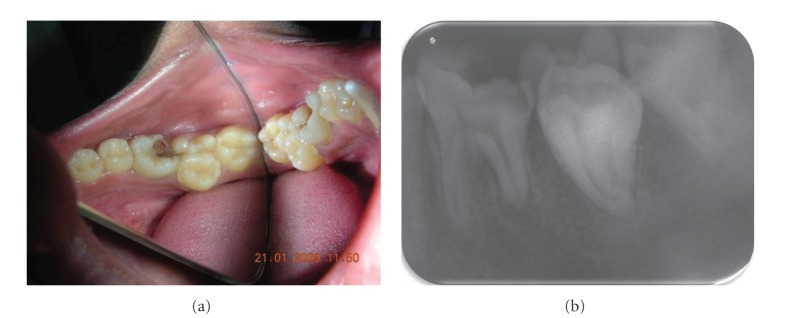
(a) Pre-operative photograph: Occlusal View. (b) Pre-operative radiograph revealing irregular morphology of tooth #20 & istal root resorption with #21.
